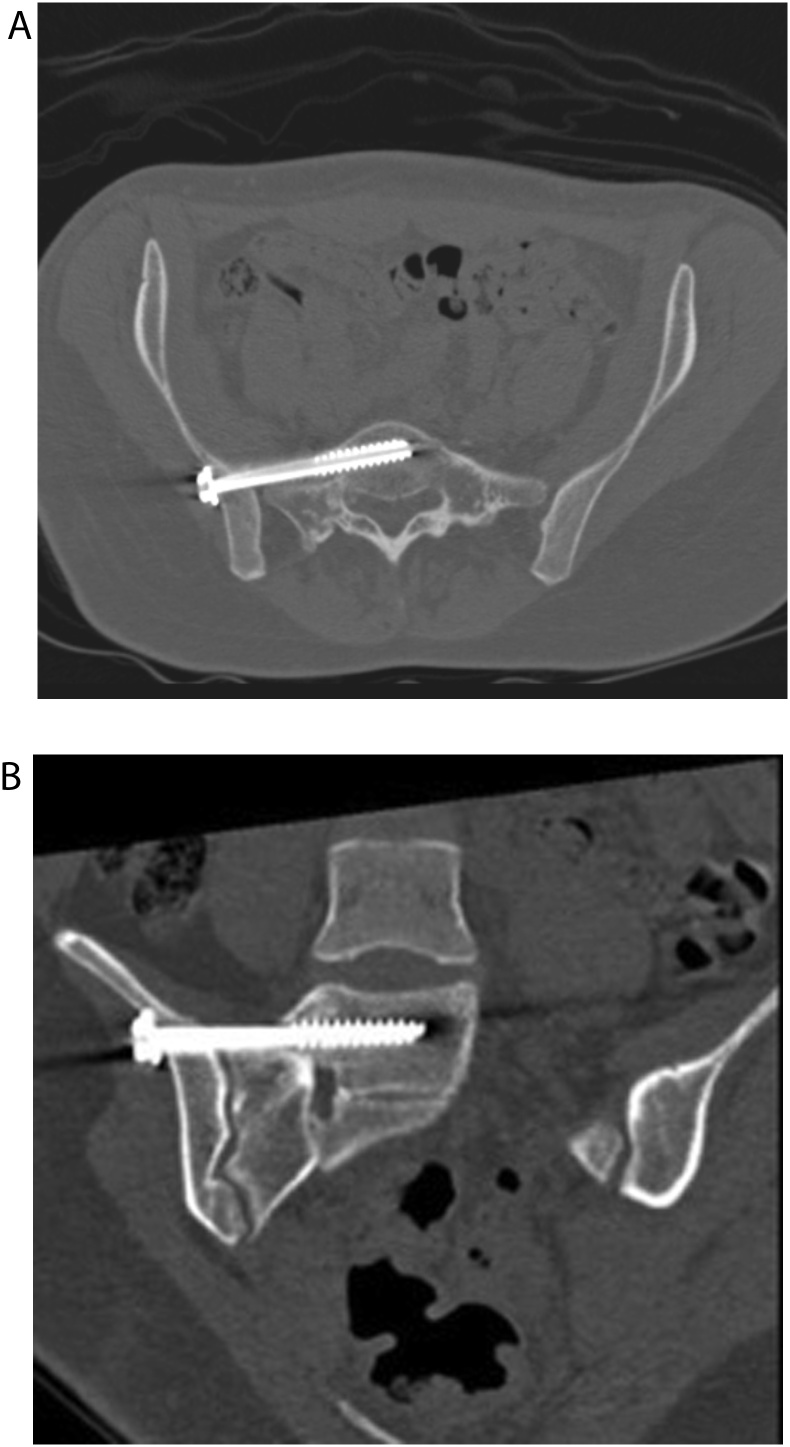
CT: (A: axial cut), and (B: coronal cut) shows an extraosseous portion of the screw perforating anterior cortex of sacral ala.
